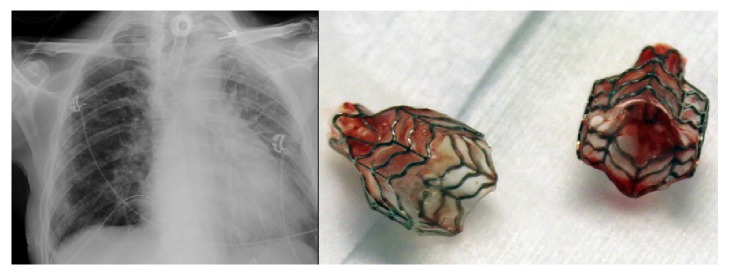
Chest X-ray (left image) after removal of the endobronchial valves (right image).
